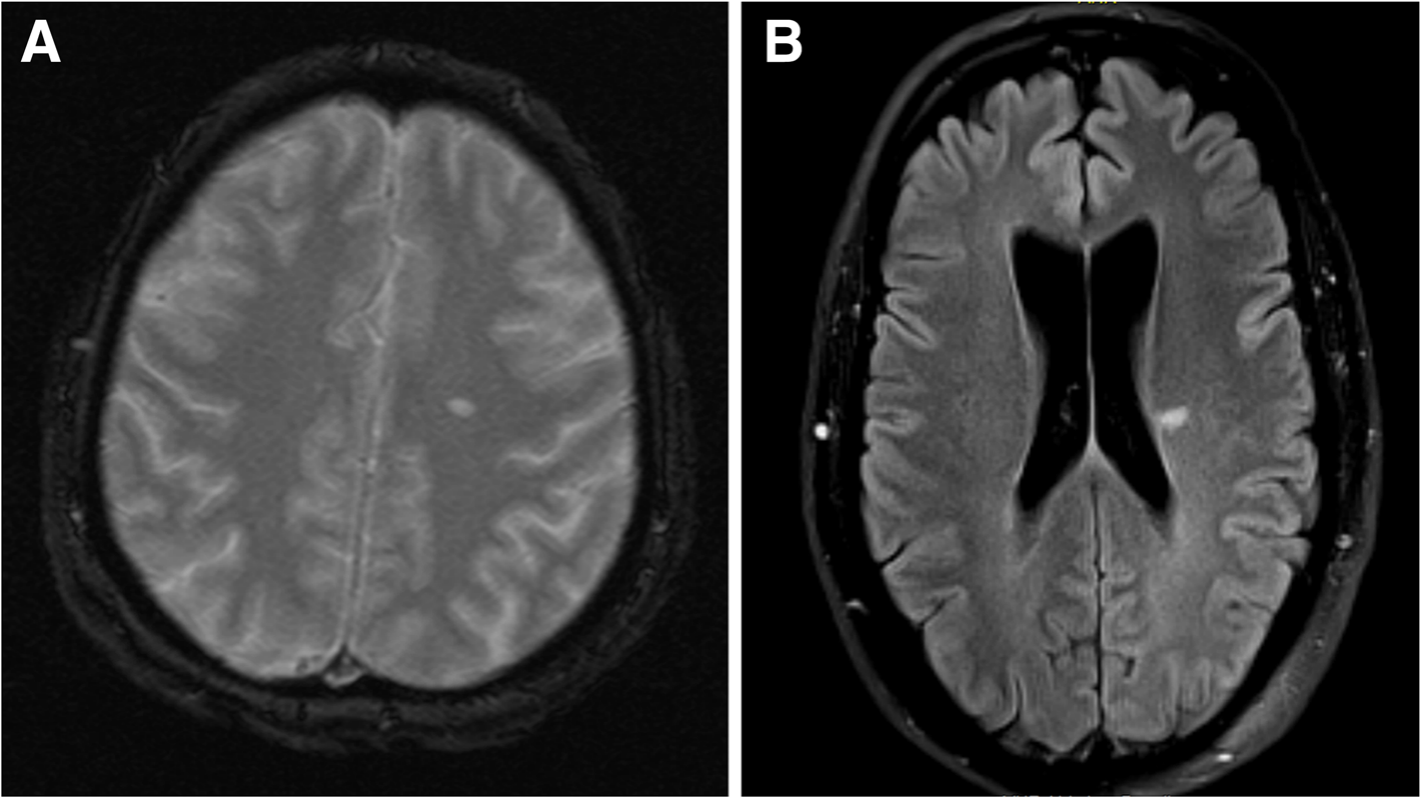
a Conventional T2-weighted imaging showing a single ischemic brain lesion in a female AF patient aged 63 years without stroke risk factors and (b) Fluid-attenuated inverse recovery (FLAIR) showing a single ischemic brain lesion in a male AF patient aged 50 years without stroke risk factors
